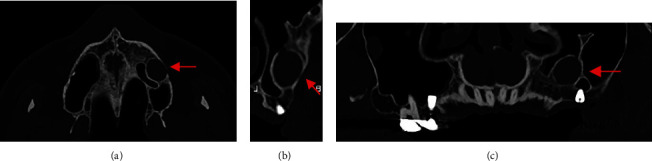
Case 1: preoperative CT scan showing round, unilocular, hypodense lesion (red arrows). (a) Axial view, (b) cross-sectional view, and (c) panoramic reconstruction view.
